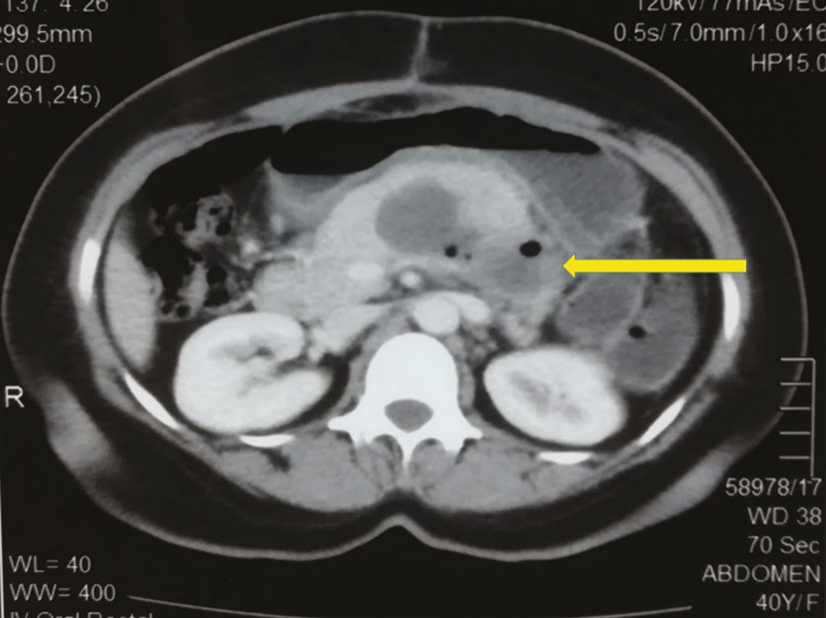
Postoperative computed tomography image showing a 7 × 4.8 × 4.9 cm mass lesion with a thin wall containing hypodense material, attached to the inferior border of the pancreas.
